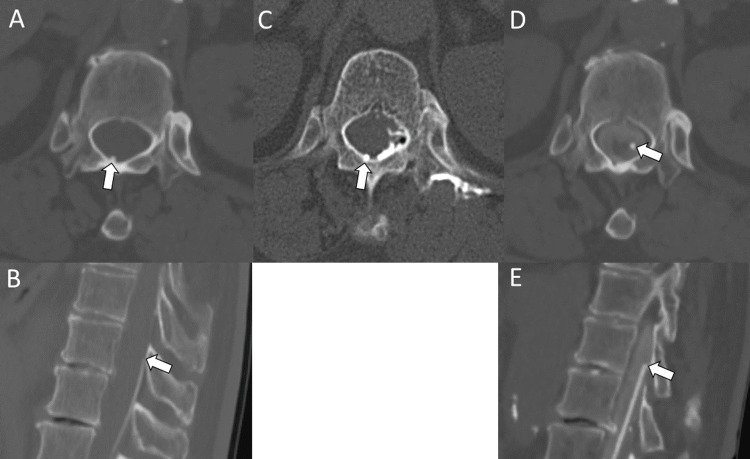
Lumbar catheter tip position before and after revision CT: computed tomography (A) Axial view and (B) sagittal view: The initial postoperative CT shows the catheter tip located at the dorsolateral aspect of the spinal canal (arrow). (C) CT at the time of shuntography also demonstrates the tip positioned at the dorsolateral aspect of the canal (arrow). (D) Axial view and (E) sagittal view: postrevision myelographic CT shows appropriate contrast spread within the subarachnoid space and the catheter tip abutting the spinal cord (arrow)
